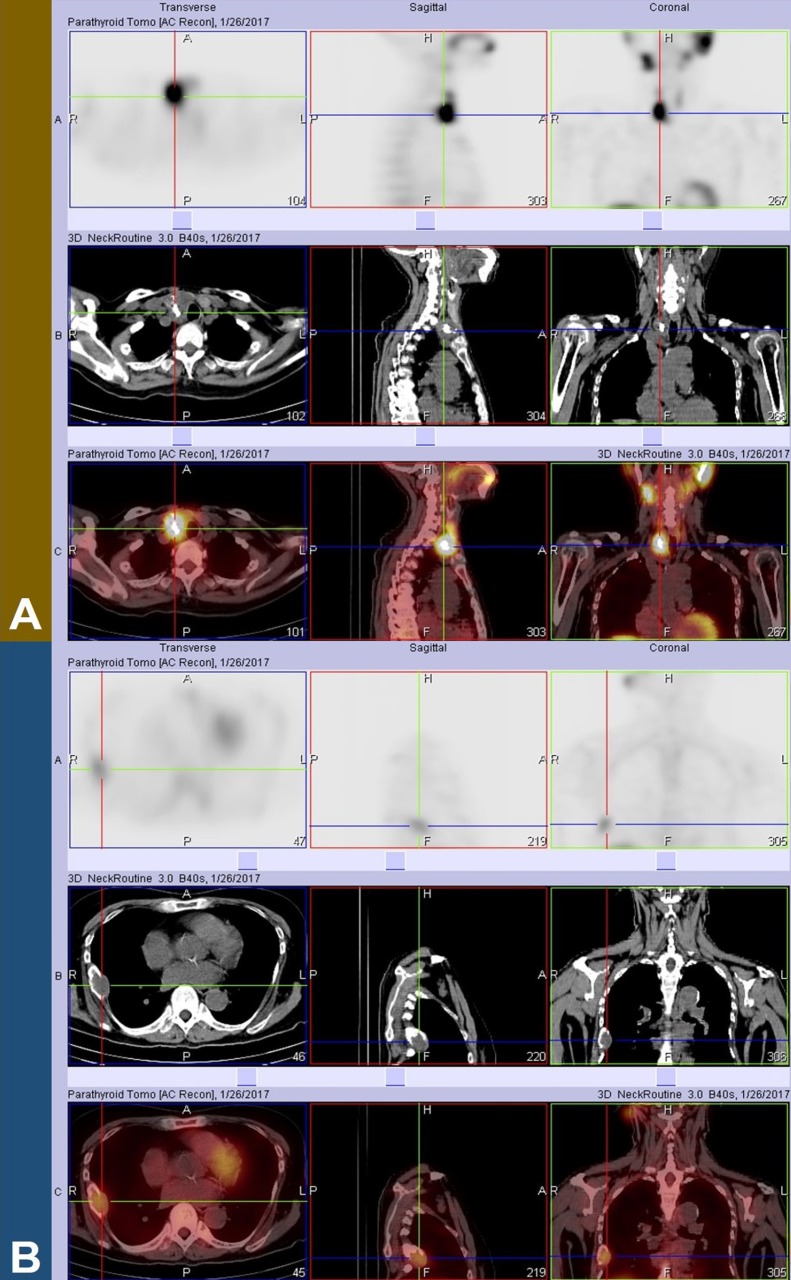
SPECT/CT revealed a sestamibi-avid lesion in the inferior pole of the right lobe (A), corresponding to a solid mass with calcification. An incidental focus of increased tracer uptake was also noted in a soft tissue mass on the 7^th^ right rib (B
